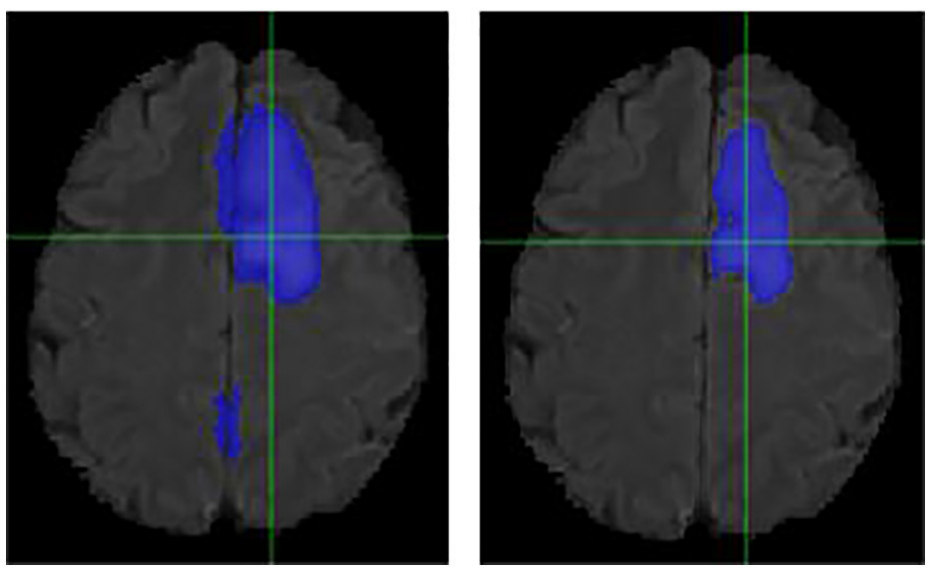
Case Two (Improved). Low-grade Glioma. Flair MRI. rMR only (Left) and Total SynMR MRI (Right) DSC of WT was improved from 0.49 to 0.88.
